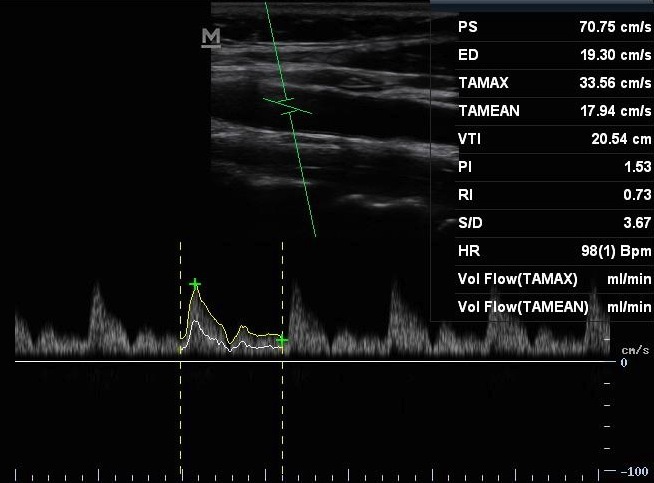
Ultrasound image of common carotid Doppler waveform and velocity time integral measurement acquired with an insonation angle of 58 degrees. The upper spectral waveform tracing (hollow arrow) represents peak flow velocity and the lower spectral waveform (solid arrow) represents mean flow velocity. Plus signs on the spectral waveform represent peak systolic velocity and end diastolic velocity.
